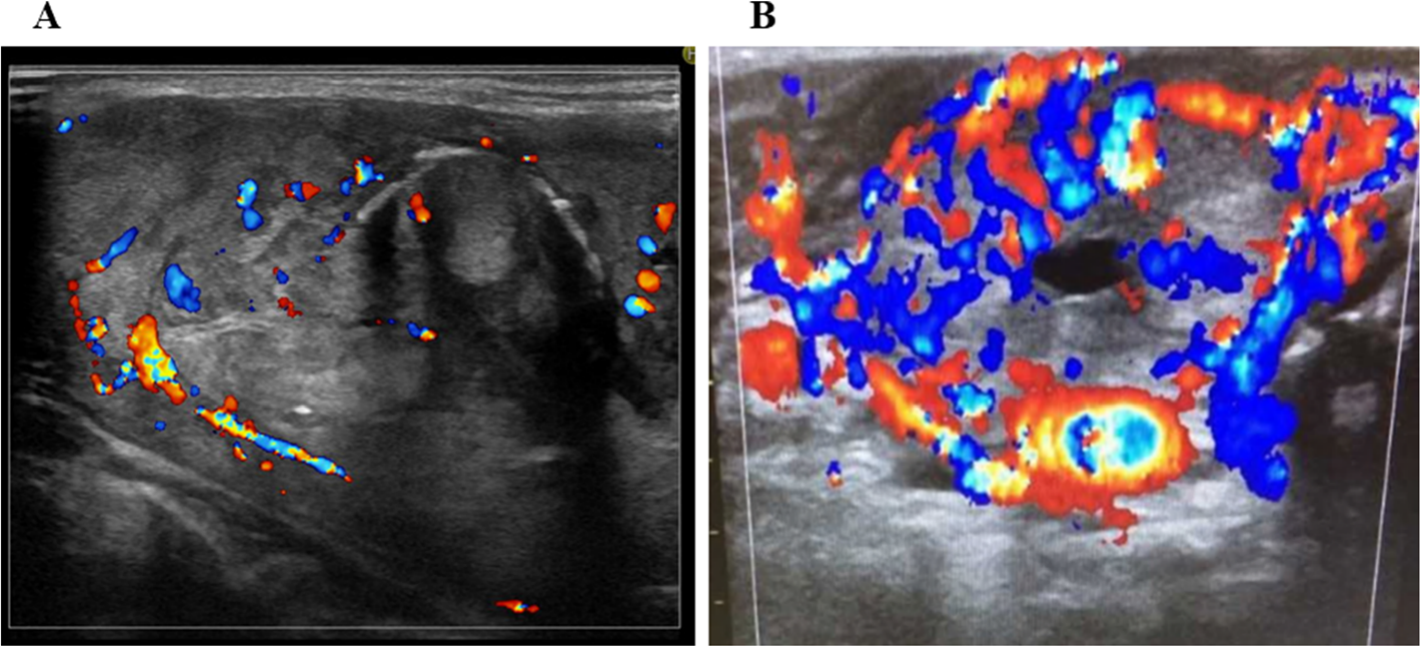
Thyroid ultrasonography. A Two years before hospitalization. A large tumour was revealed in the right lobe with calcification and slight blood flow. B At the time of hospitalization. Diffuse enlargement of the right lobe with increased blood flow was revealed
